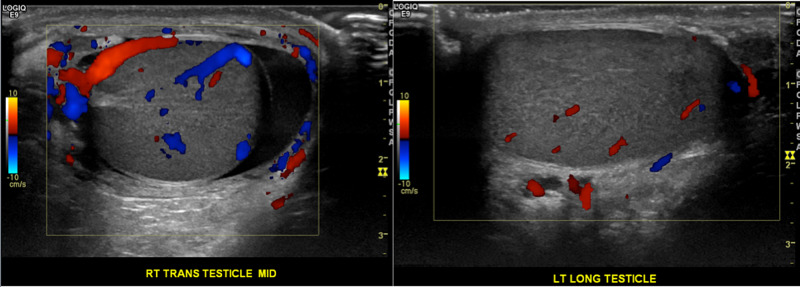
Color Doppler ultrasonography of the bilateral testes Doppler ultrasonography demonstrates normal color flow across both testes.
